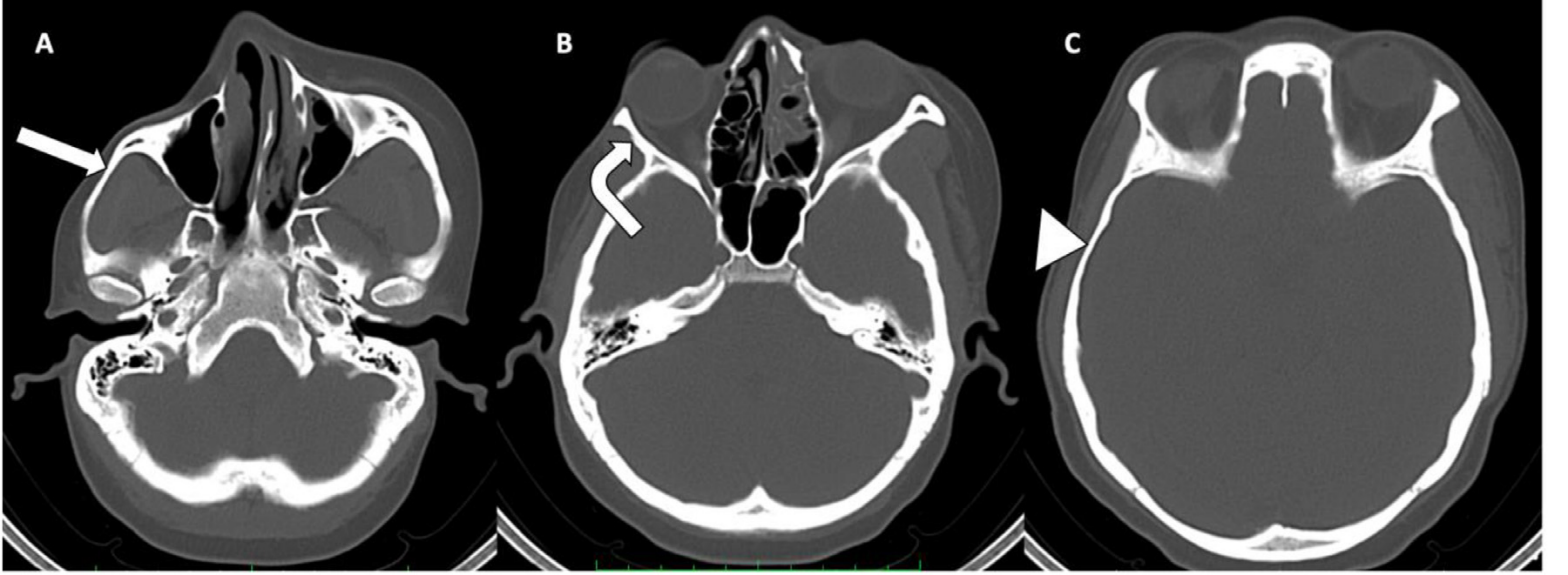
(A-C) Axial CT scans of the head (bone window) showing atrophy of the right zygoma and zygomatic process (*white bloack arrow*) (A), the right greater wing of sphenoid bone (*white bent arrow*) (B) and squamous part of the right temporal bone (*white arrowhead*) (C).
